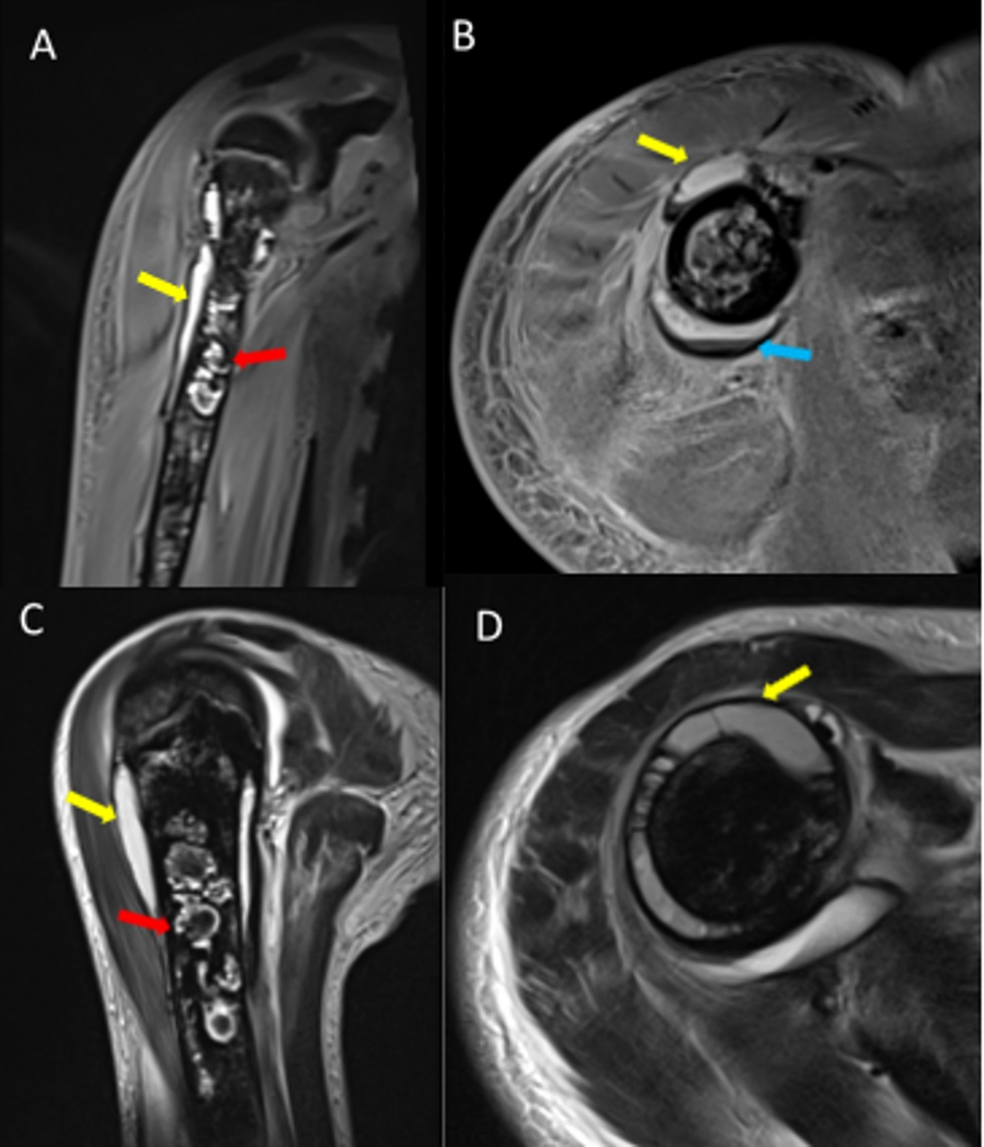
MRI of the right humerus. (A) Coronal T1, (B) axial T1 fat-suppressed, (C) sagittal T2, and (D) axial T2 images showing hyperintense localized subperiosteal fluid collections (yellow arrows) with fluid-debris levels (blue arrow). Ill-defined cystic changes in the proximal and distal metaphysis of the right humerus are likely due to iron deposition, chelation, and red marrow conversion (red arrows).
